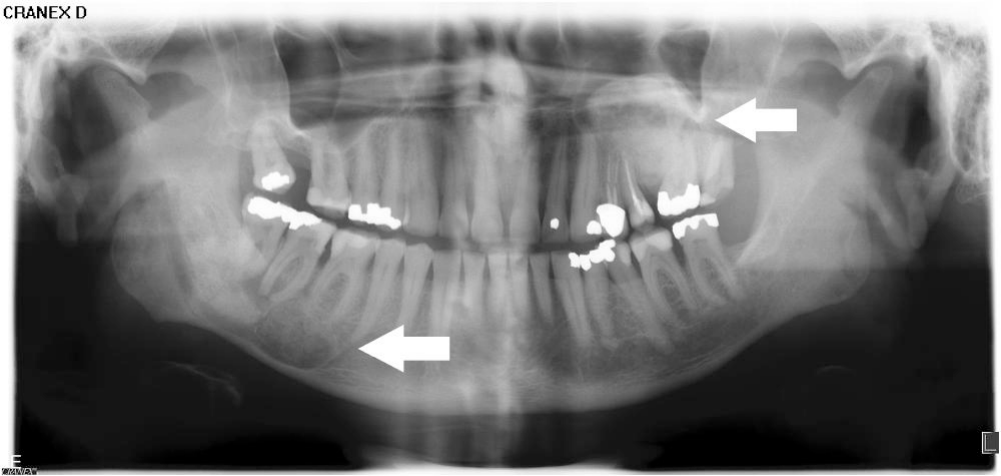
Panoramic x-ray of the jaws from 2014 showing an intraosseous lesion in the lower right mandible and upper left maxilla.
